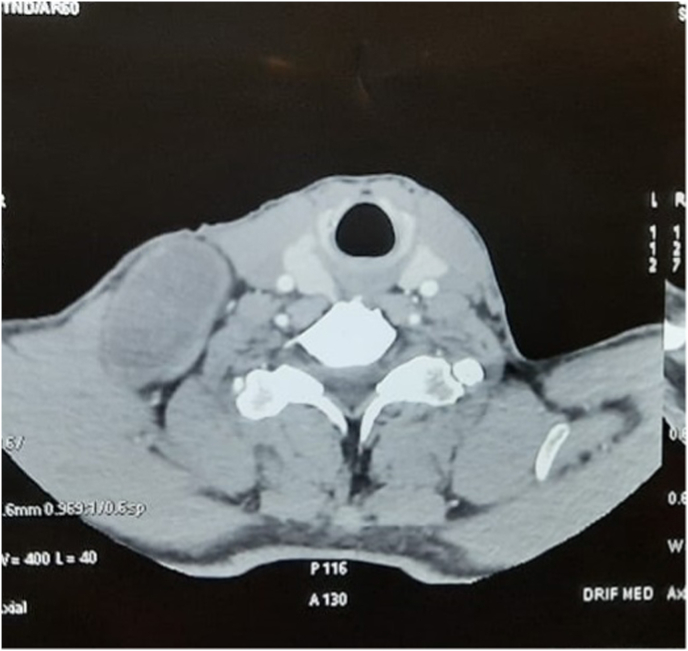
CT scan revealing a large well-defined mass located in the right supraclavicular region.
